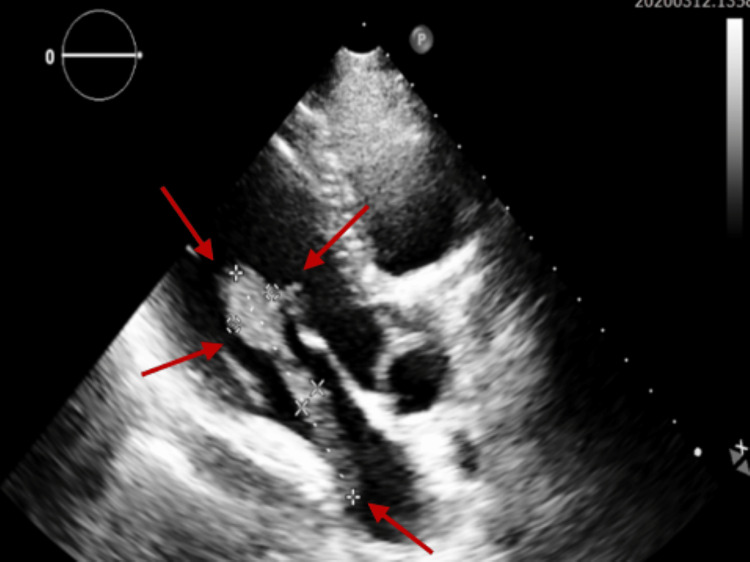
Transthoracic echocardiography modified long-axis view, showing an elongated mass (1.6 cm x 0.6 cm x 6.3 cm) protruding into the left ventricle during diastole
